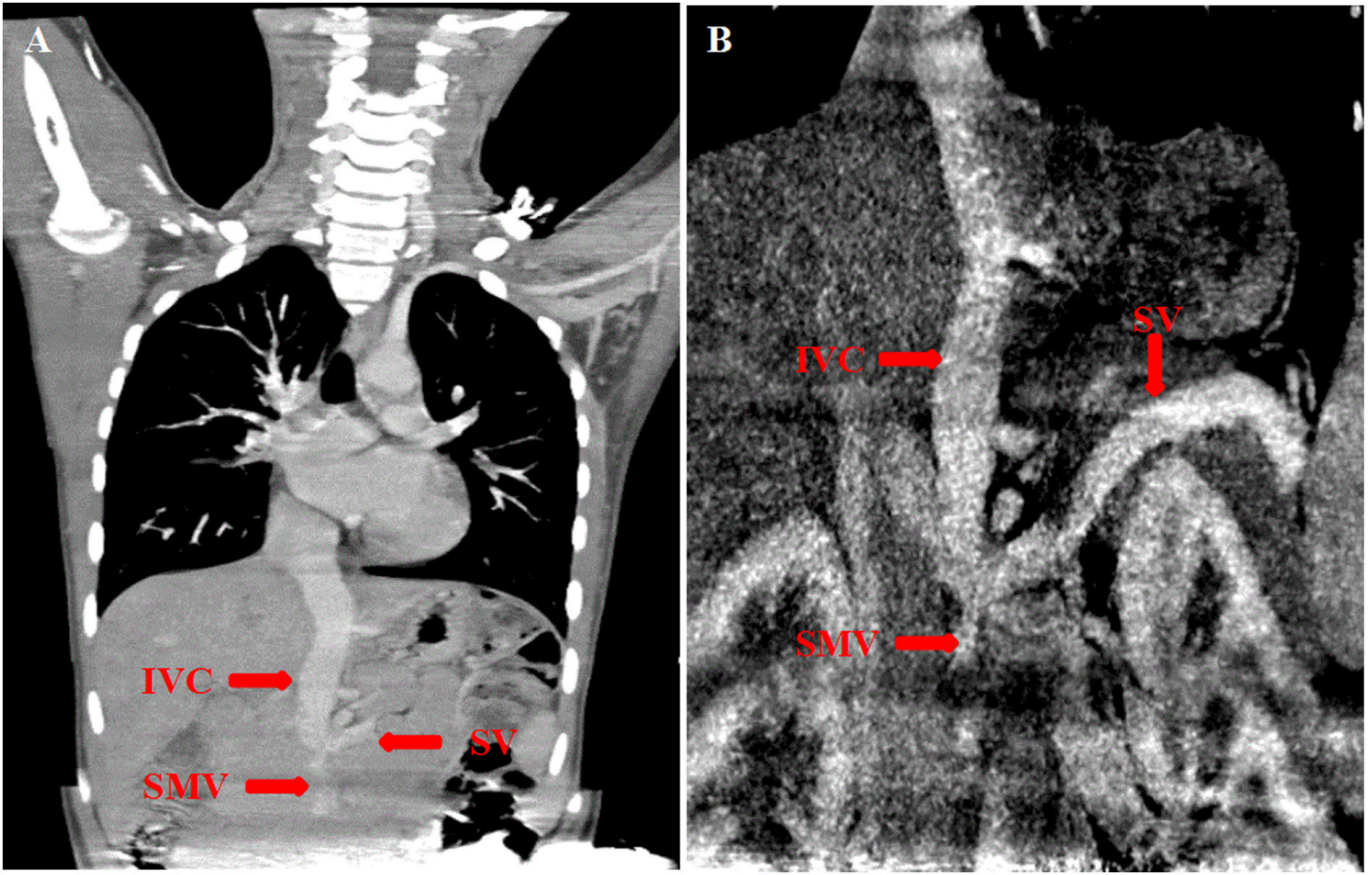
(A,B) Computer tomography angiography (CTA) showed the splenic vein (SV) joined the superior mesenteric vein (SMV) and then flowed upward into the inferior vena cava (IVC) directly.
